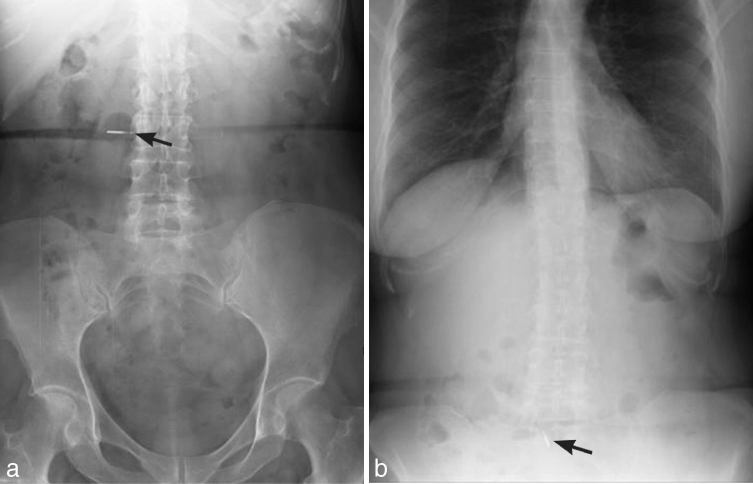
Supine abdominal anteroposterior radiographs. At presentation (a), a linear opaque right upper quadrant object, a dental bit (arrow), was presumed to be within the pre-pyloric region of the stomach or in the first portion of the duodenum. A follow-up radiograph performed on the same date (b) demonstrated migration to the pelvis.
